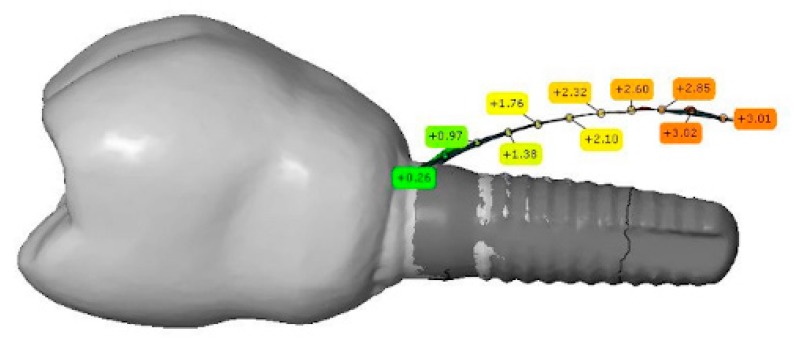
Matching between the a priori information and the ultrasound data. The numbers describe the thickness of bone above the implant surface. The color shades are defined as follows: orange corresponds to a thickness of 3 mm and green to a thickness of zero.
